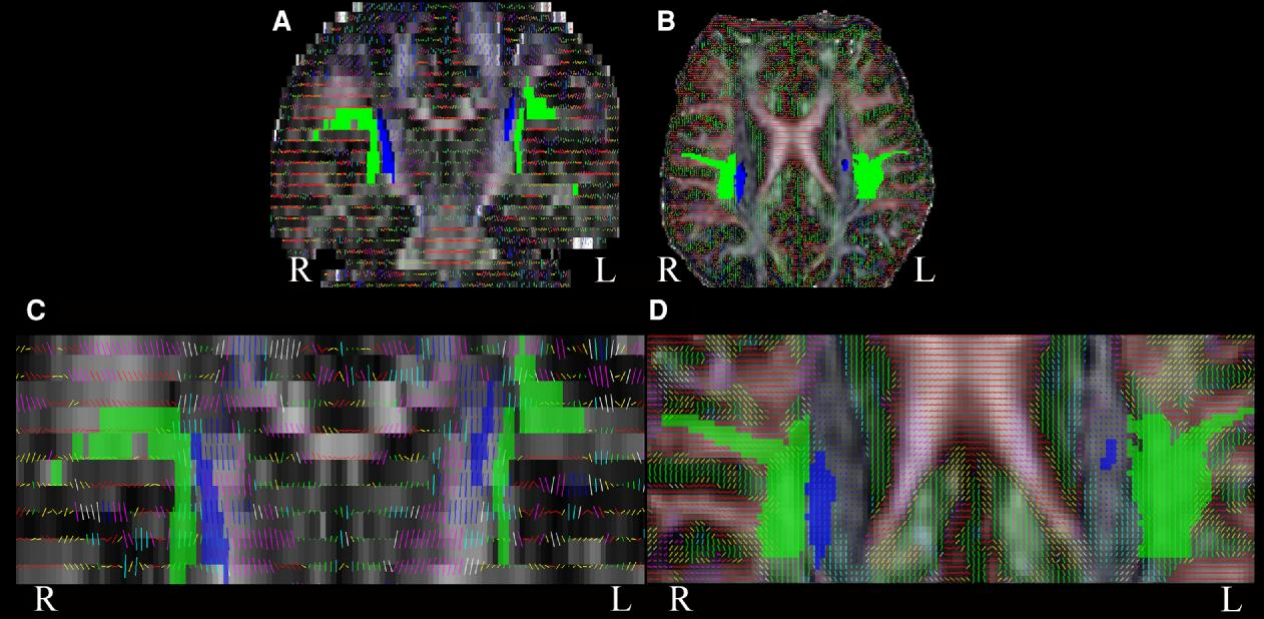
Eigenvectors overlying deviated CST in ALS. Coronal (A) and axial (B) views of a brain fractional anisotropy MRI from an ALS patient with corticospinal tract (CST) hyperintensity (ALS-CST+), show tractography-derived CST (blue) and superior longitudinal fasciculus (SLF, green) at the level of centrum semiovale at top of lateral ventricle. Higher magnification coronal (C) and axial (D) views, where clearly seen overlying eigenvectors show virtual CST fibres in the right hemisphere entering the SLF and becoming truncated, while those in the left hemisphere do not.
